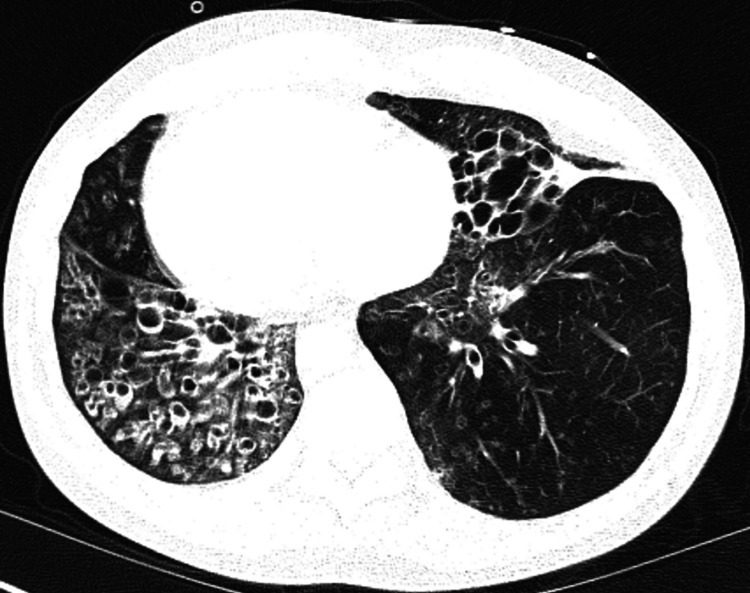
CT scan of the chest demonstrating severe bronchiectasis.
